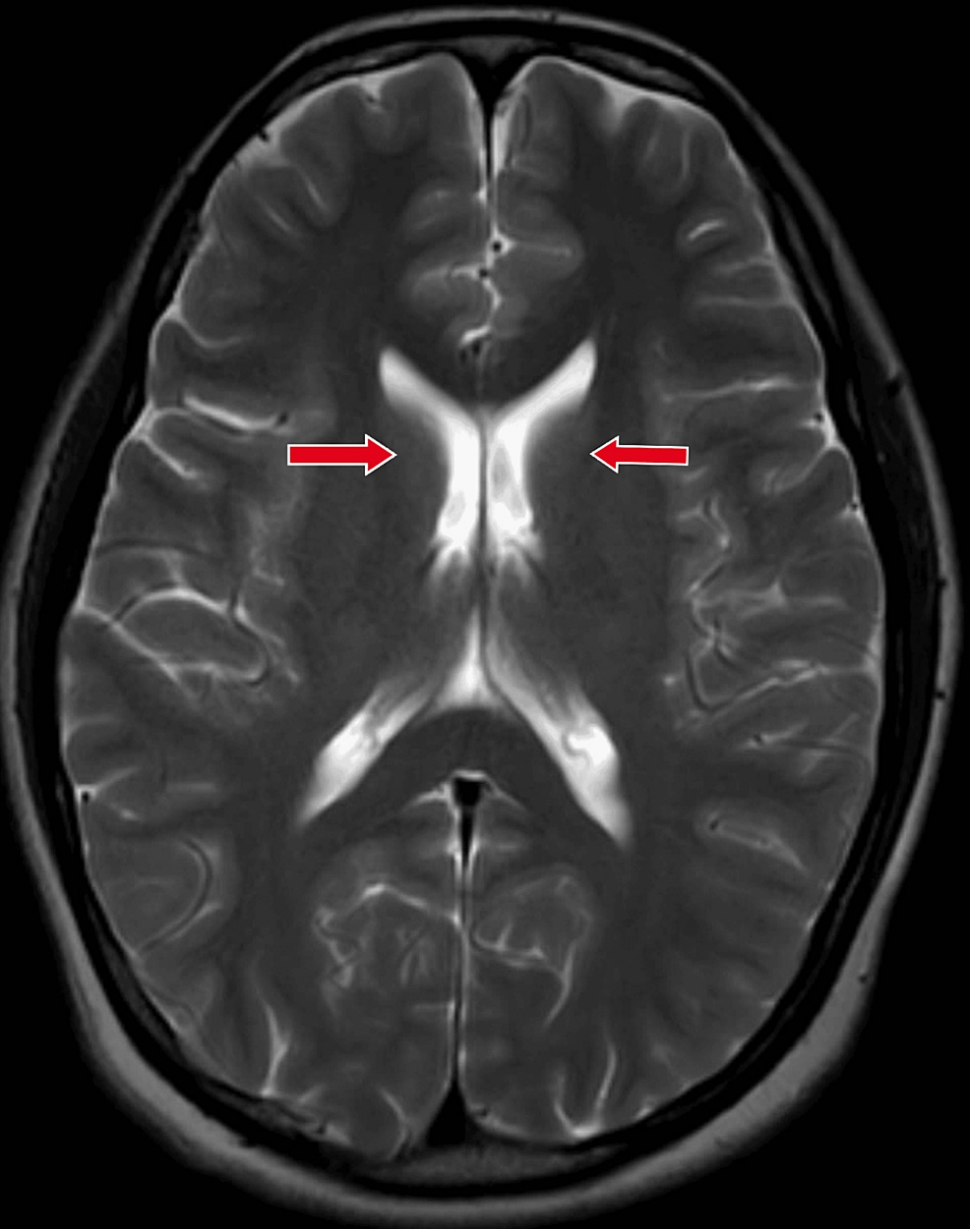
MRI T2-weighted axial section of brain suggesting normal imaging study. Magnetic resonance imaging (MRI) of the brain with normal appearing non-atrophied bilateral caudate nucleus with no abnormal hypo- or hyper-intensities shown with red arrows.
